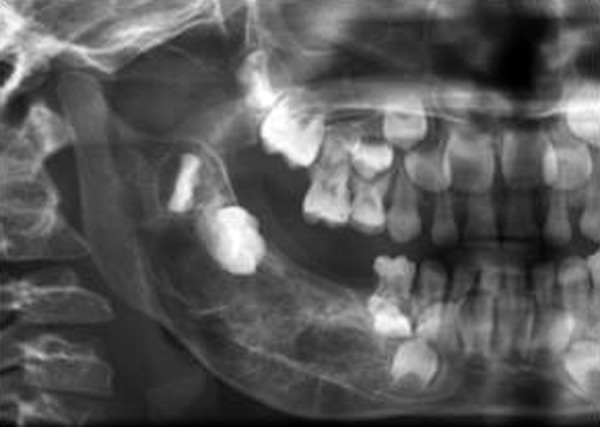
Cropped post-treatment panoramic radiograph shows uniform expansion with cortical regeneration throughout the lower border of the mandible. Mixed radiodensity seen in internal structure
